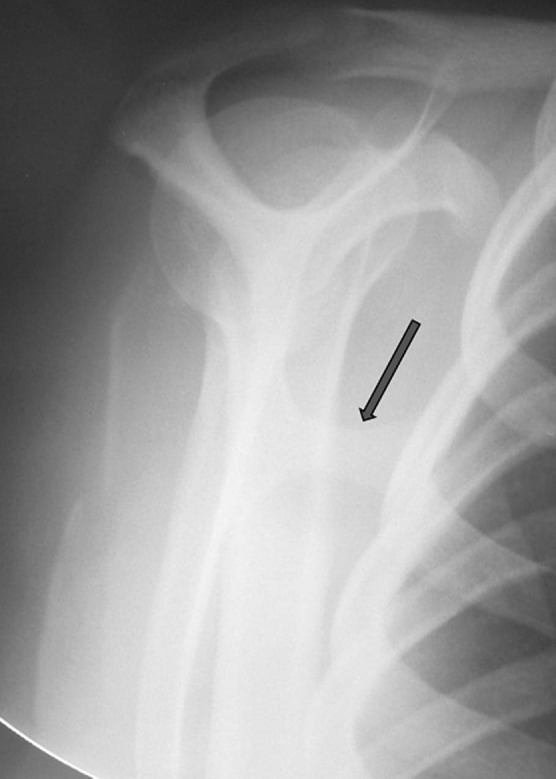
standard radiography showing a pedunculated bony structure extruding from the right scapula
